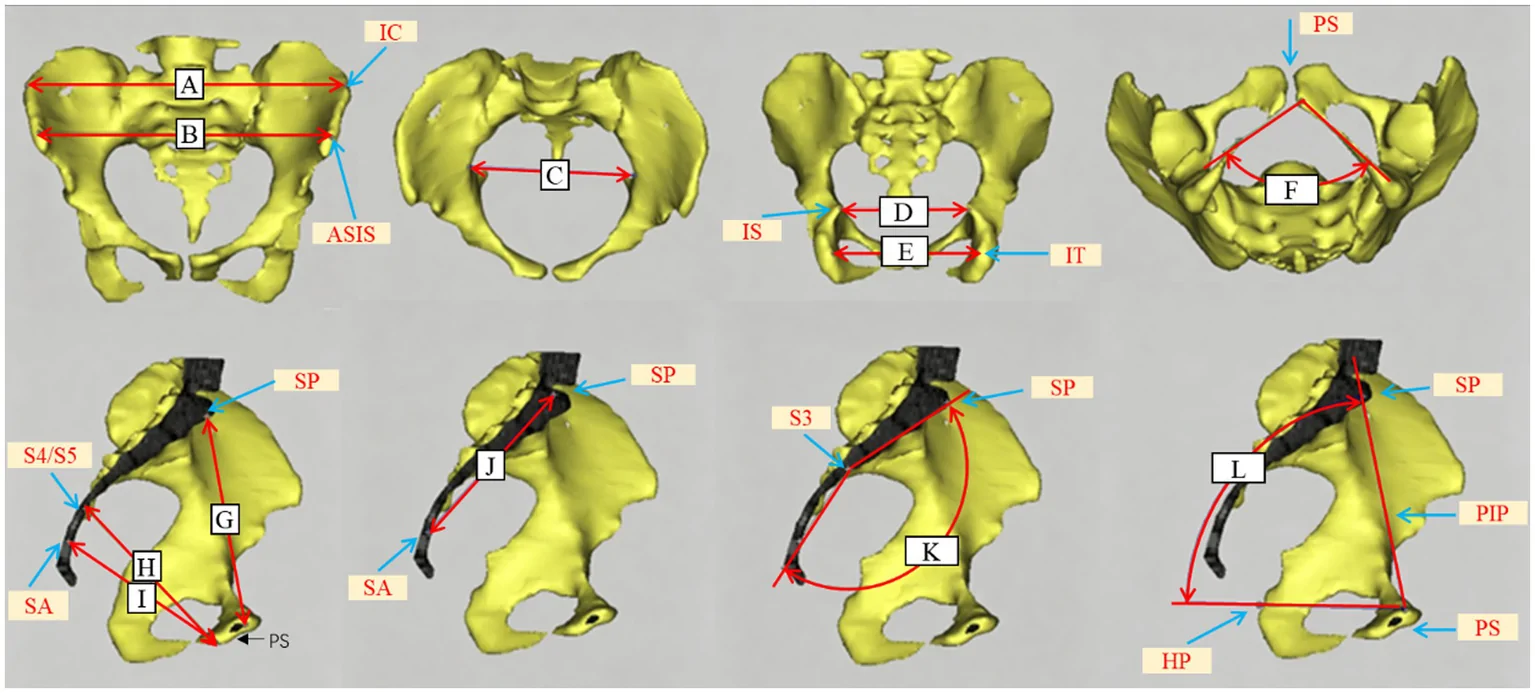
Anatomical landmarks and procedures used for pelvic measurements on MRI-based three-dimensional pelvic models. Red double-headed arrows, lines, and arcs indicate the measured distances or angles, whereas blue arrows indicate the corresponding anatomical landmarks and reference planes. (A) Intercristal diameter, measured as the maximum distance between the most lateral points of the bilateral iliac crests. (B) External interspinous diameter, measured between the bilateral anterior superior iliac spines. (C) Transverse diameter of the pelvic inlet, measured as the maximum transverse distance across the pelvic inlet. (D) Transverse diameter of the midpelvis, measured between the bilateral ischial spines. (E) Transverse diameter of the pelvic outlet, measured between the inner margins of the bilateral ischial tuberosities. (F) Subpubic angle, defined as the angle formed by the bilateral ischiopubic rami. (G) Anteroposterior diameter of the pelvic inlet, measured from the sacral promontory to the posterior superior margin of the pubic symphysis. (H) Anteroposterior diameter of the midpelvis, measured from the S4–S5 level to the inferior margin of the pubic symphysis. (I) Anteroposterior diameter of the pelvic outlet, measured from the sacral apex to the inferior margin of the pubic symphysis. (J) Sacral length, measured as the straight-line distance from the sacral promontory to the sacral apex. (K) Sacral curvature angle, defined as the angle formed by the sacral promontory, the midpoint of the anterior surface of S3, and the sacral apex, with the S3 point as the vertex. (L) Pelvic inclination, defined as the angle between the pelvic inlet plane and the horizontal plane. IC, iliac crest; ASIS, anterior superior iliac spine; IS, ischial spine; IT, ischial tuberosity; PS, pubic symphysis; SP, sacral promontory; SA, sacral apex; S3, third sacral vertebra; S4/S5, S4–S5 level; PIP, pelvic inlet plane; HP, horizontal plane.
